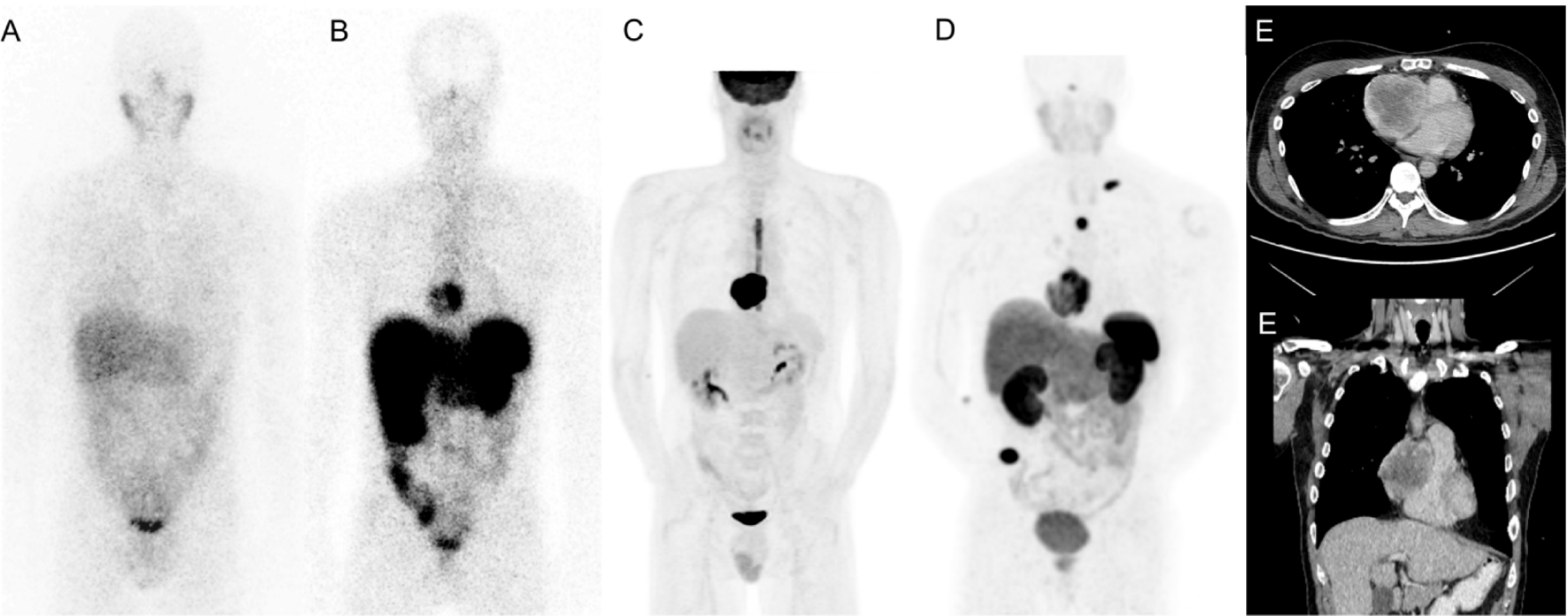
Staging imaging; ^123^I-MIBG scintigraphy (A), ^111^In-Pentetreotide scintigraphy (B), ^18^F-FDG PET/CT (C), ^68^Ga-DOTATATE SPECT-CT PET/CT (D), Contrast-enhanced CT (E).
